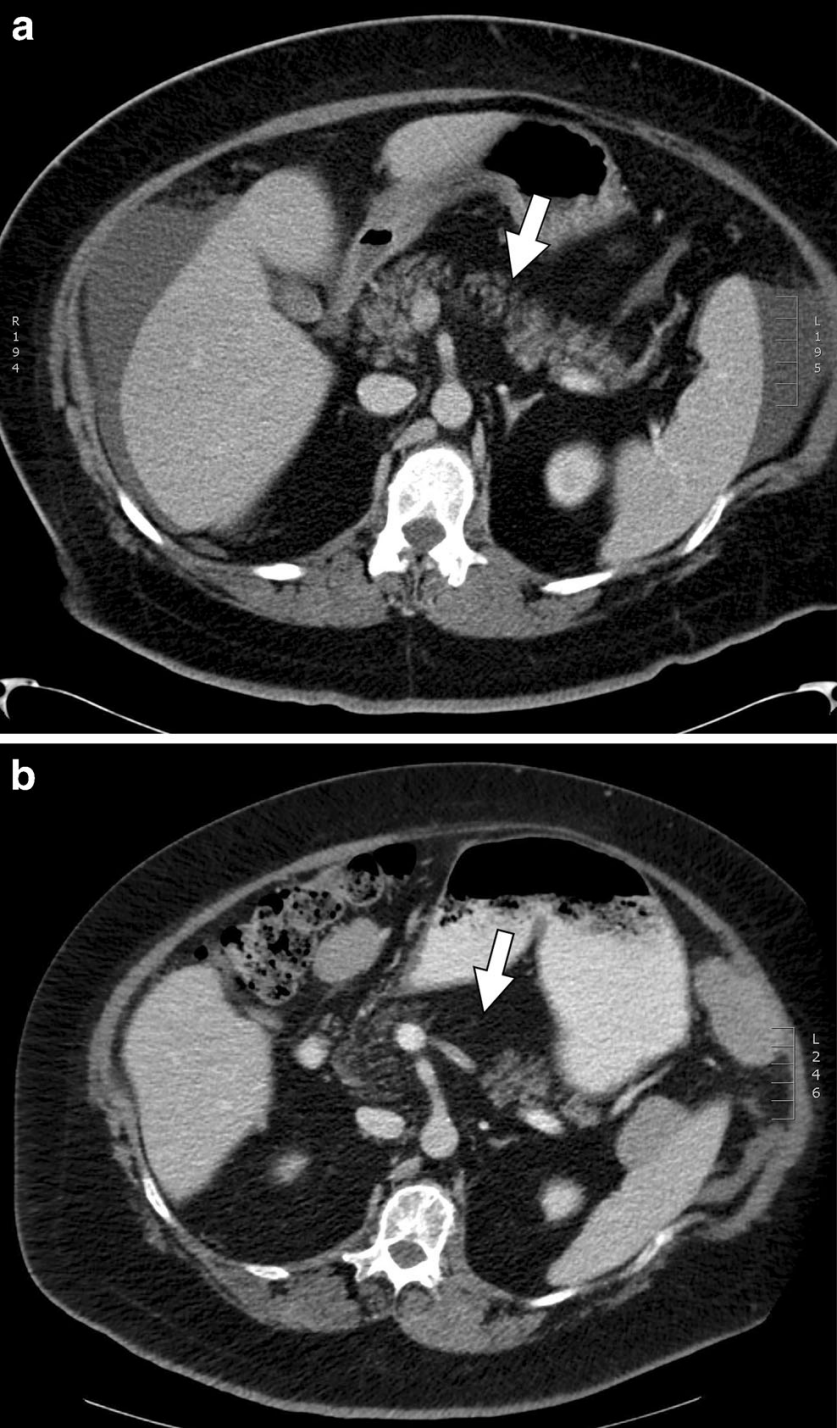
A 51-year-old female with stage IIIc ovarian cancer treated with bevacizumab. a Axial contrast-enhanced CT of the pancreas pre-chemotherapy with normal findings (*arrow*). b Repeat axial contrast-enhanced CT 11 months after initiation of chemotherapy shows marked pancreatic atrophy as well as fatty replacement (*arrow*)
